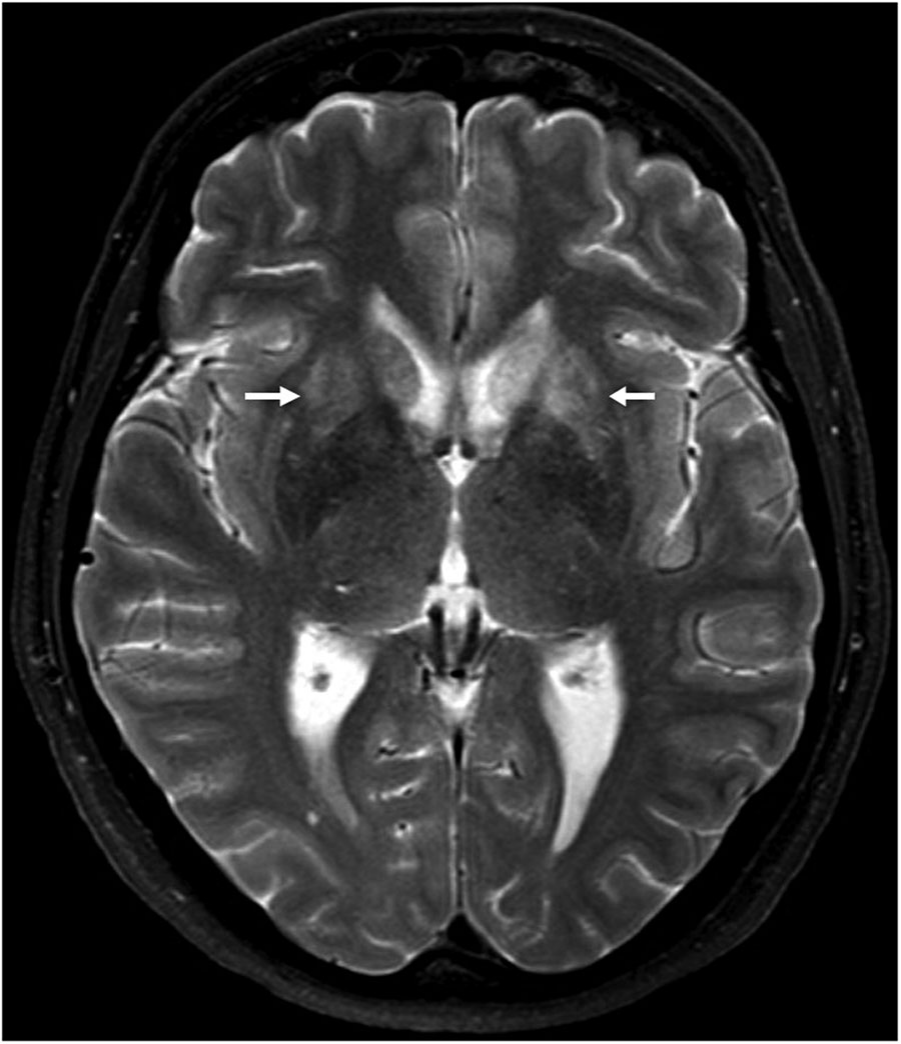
Cranial magnetic resonance imaging of case 1. The typical affection of the basal ganglia (arrows) as found in acute BoDV-1 encephalitis [10] is shown in this case of a probable BoDV-1 encephalitis according to the case definition criteria [7]. Transversal T2-weighted image.
